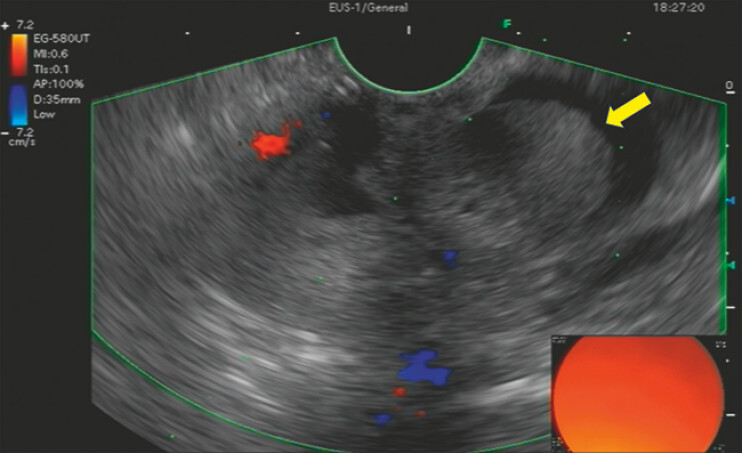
Endoscopic ultrasonography demonstrated an oval and non-shadowing polypoid mass (yellow arrow) in the distal CBD. Abbreviation: CBD, common bile duct.
